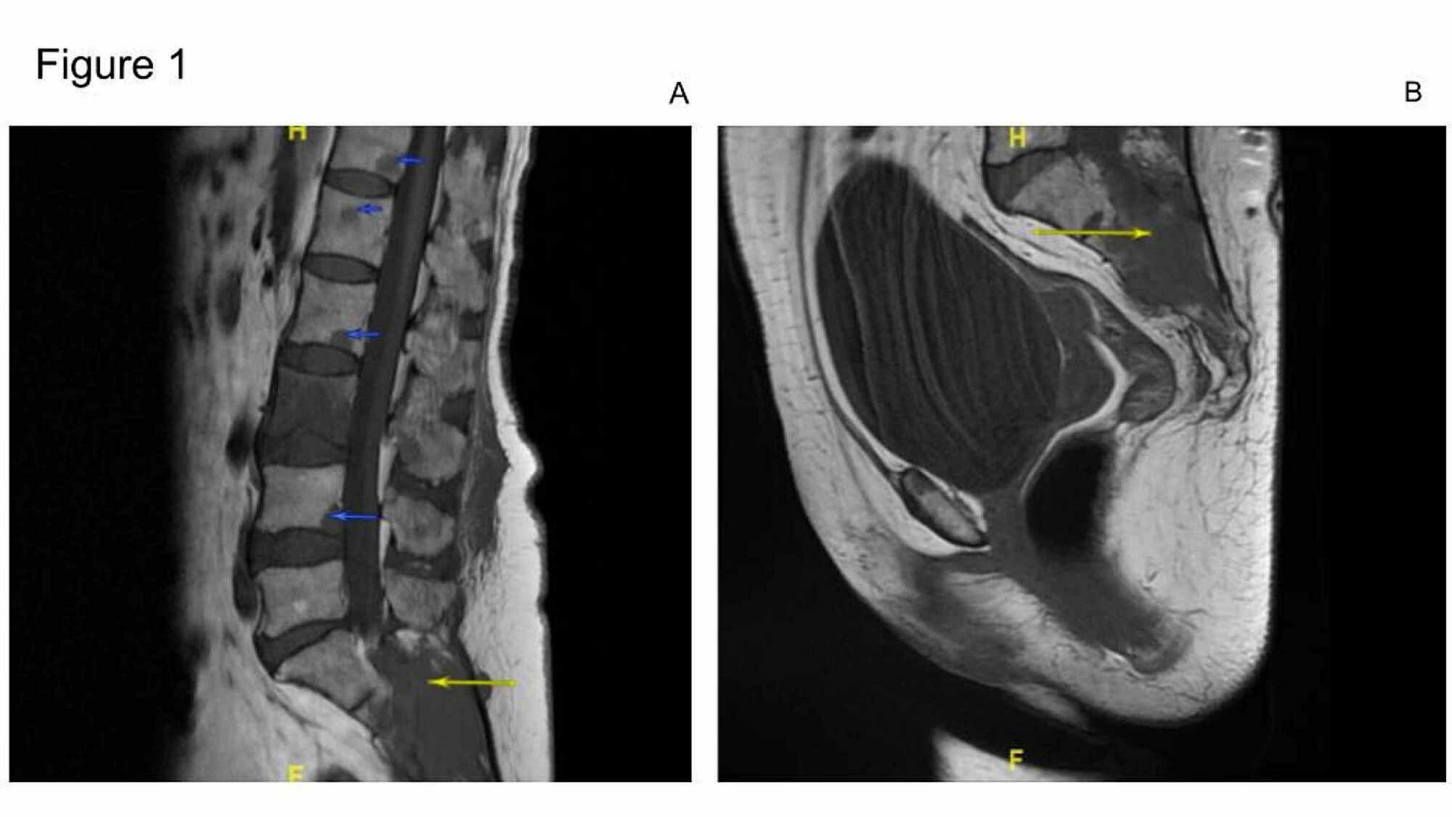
Contrast-enhanced magnetic resonance imaging (MRI) of the lumbar spine and sacrum Contrast-enhanced sagittal MRI images showing innumerable destructive lesions throughout the lumbar spine and sacrum (yellow arrows, Figure 1A) with a 5.6 x 3.5 cm destructive lesion involving the posterior S1, S2, and S3 segments of the sacrum with extension into the sacral spinal canal (blue arrows, Figure 1A-B).
